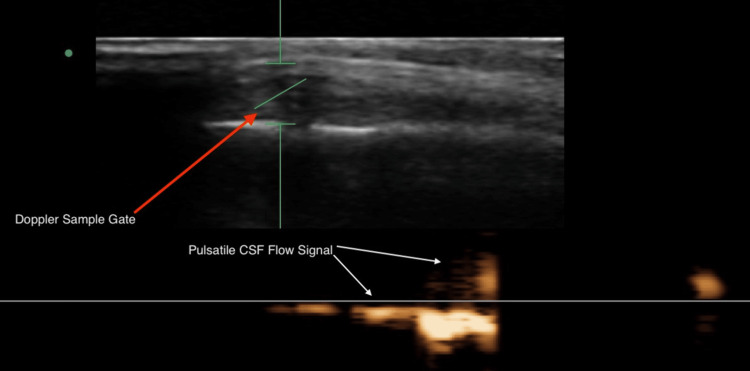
Pulse-wave Doppler ultrasound demonstrating pulsatile cerebrospinal fluid flow (white arrows) within the ventriculoperitoneal shunt. The Doppler sample volume is positioned over the shunt tubing (red arrow), generating a spectral waveform consistent with dynamic CSF flow. CSF: cerebrospinal fluid
